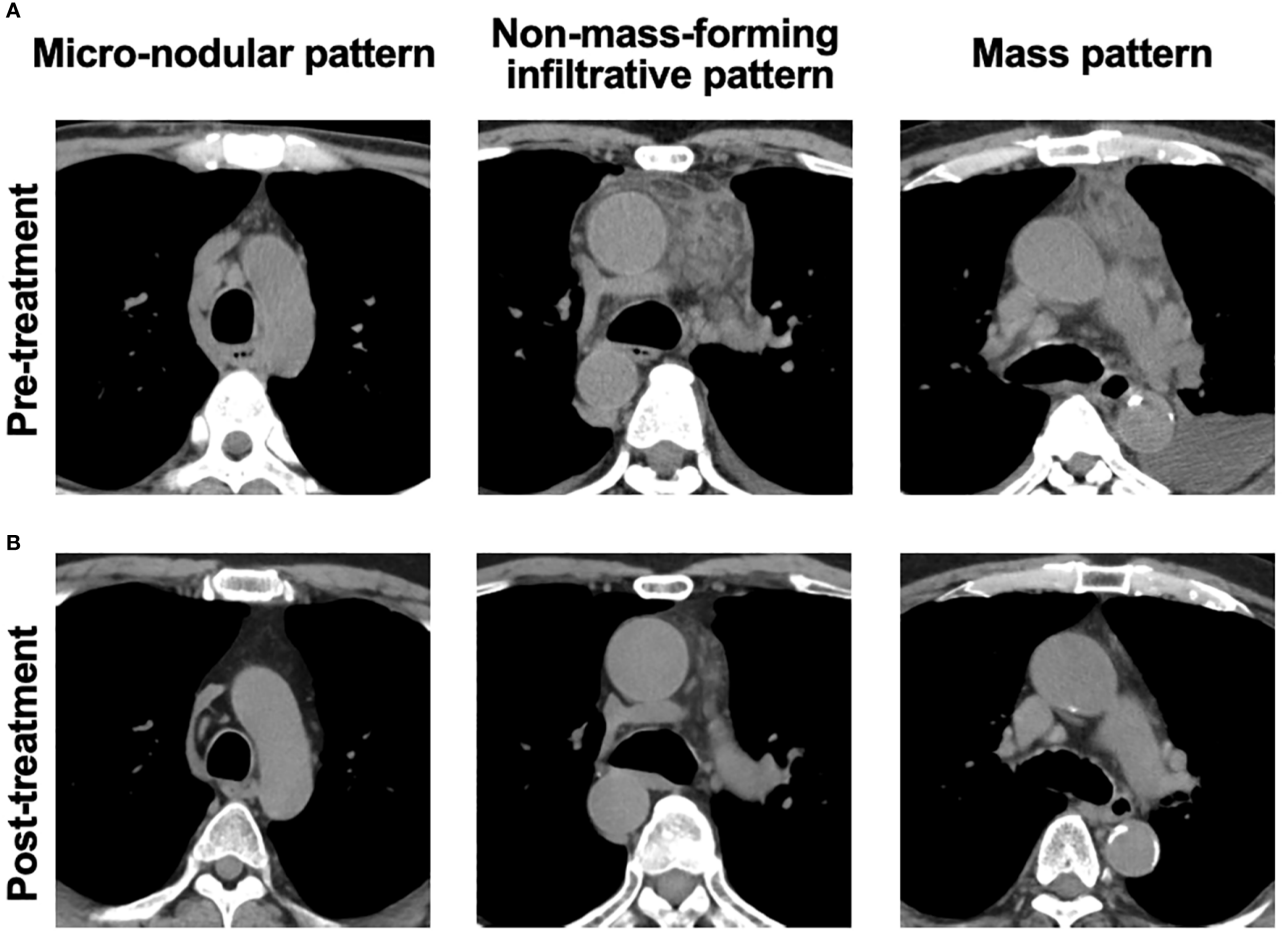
Representative images of abnormalities in anterior mediastinum lesions. (A) Representative pre-treatment CT images showing three types of anterior mediastinal abnormalities: the micro-nodular pattern (idiopathic multicentric Castleman disease-not otherwise specified [iMCD-NOS]), the non-mass-forming infiltrative pattern (idiopathic multicentric Castleman disease-TAFRO subtype [iMCD-TAFRO]), and the mass pattern (TAFRO syndrome). (B) Post-treatment CT images showing improvement in each respective abnormality.
